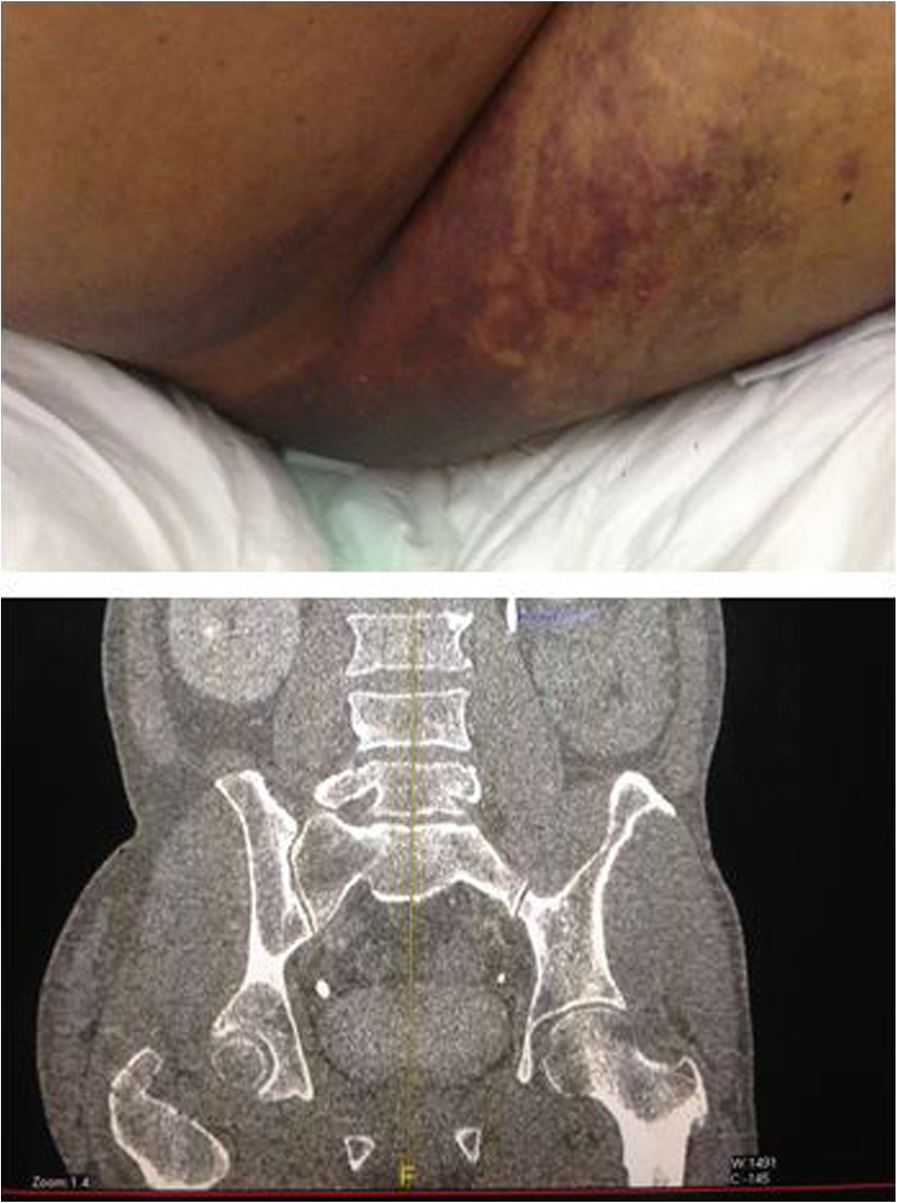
Morel Lavallee lesion (common site) and Coronal CT view of the lesion
